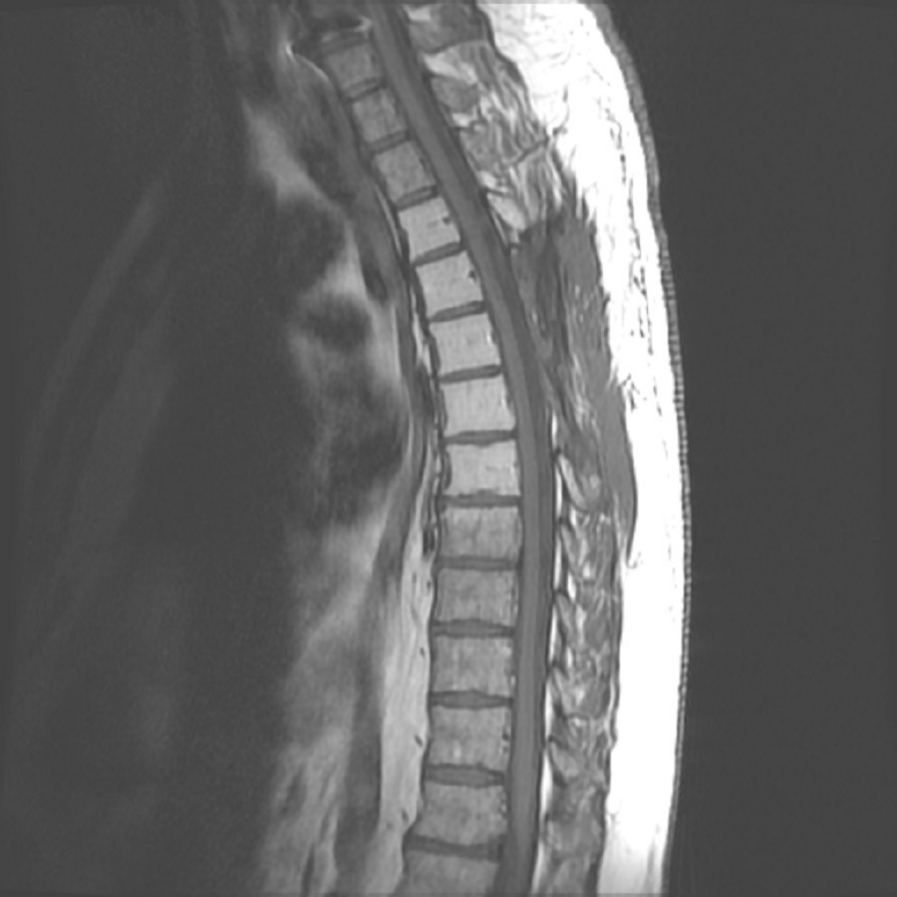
Postoperative T1-weighted images with contrast. T1-weighted sagittal images with gadolinium of the thoracic spine MRI show postoperative changes with no evidence of a residual mass at the T4-T6 levels. There is a decompression of the spinal cord.
